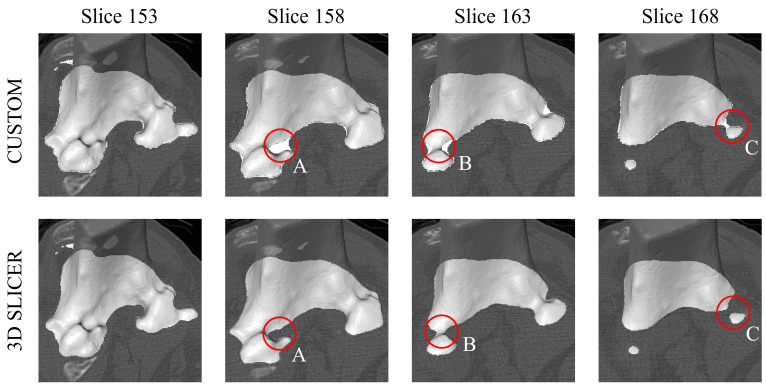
Label generation comparison. (First row): Custom algorithm; (Second row): 3D Slicer-based algorithm. For each slice of the CT, its corresponding bone reference surface and the segmentation labels (in white over the CT) are shown. A, B, and C circled areas highlight major differences in critical regions for the two approaches.
